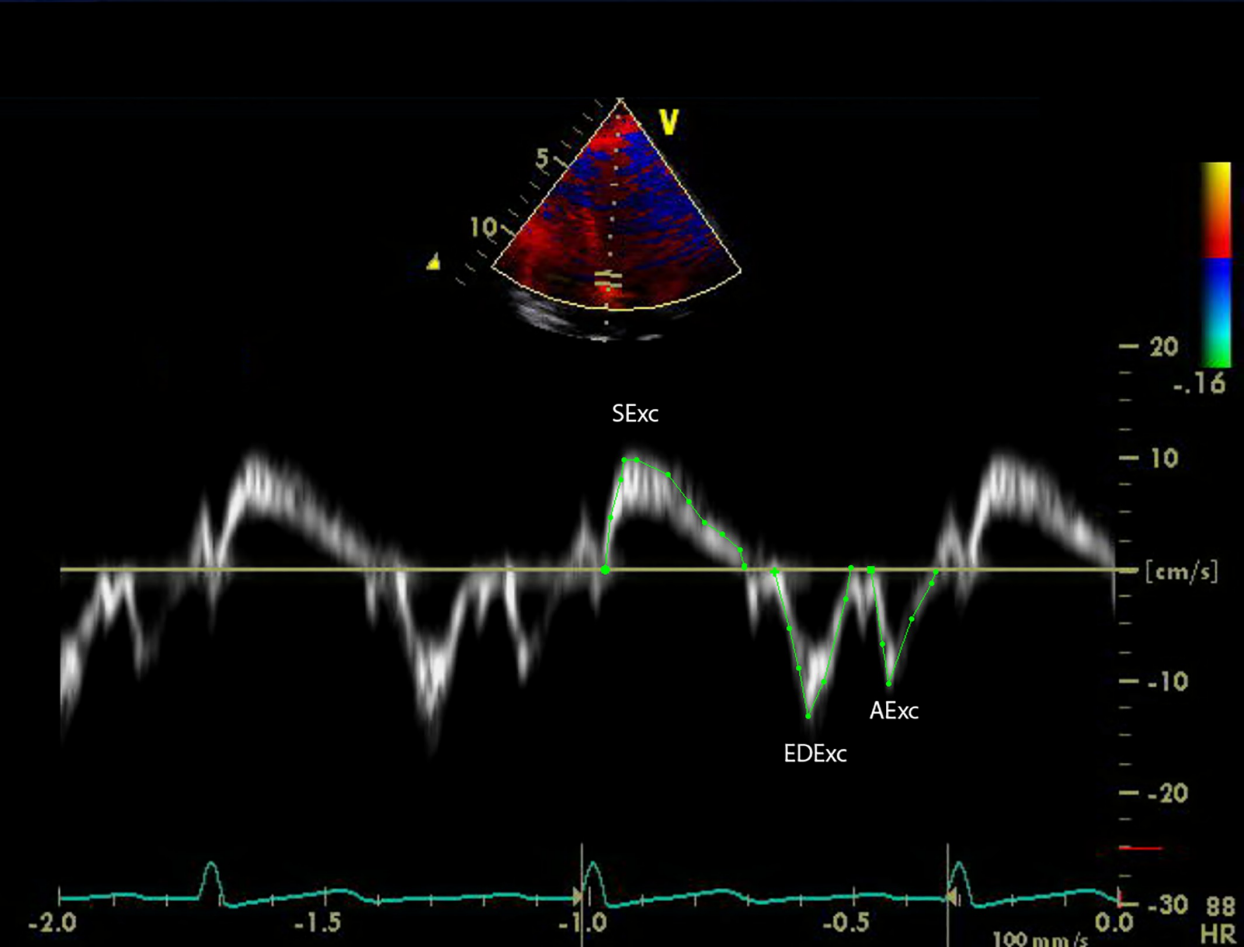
Example of the method for tracing the velocity time integrals of the systolic, early diastolic and atrial contraction tissue Doppler signals from the mitral annulus.
